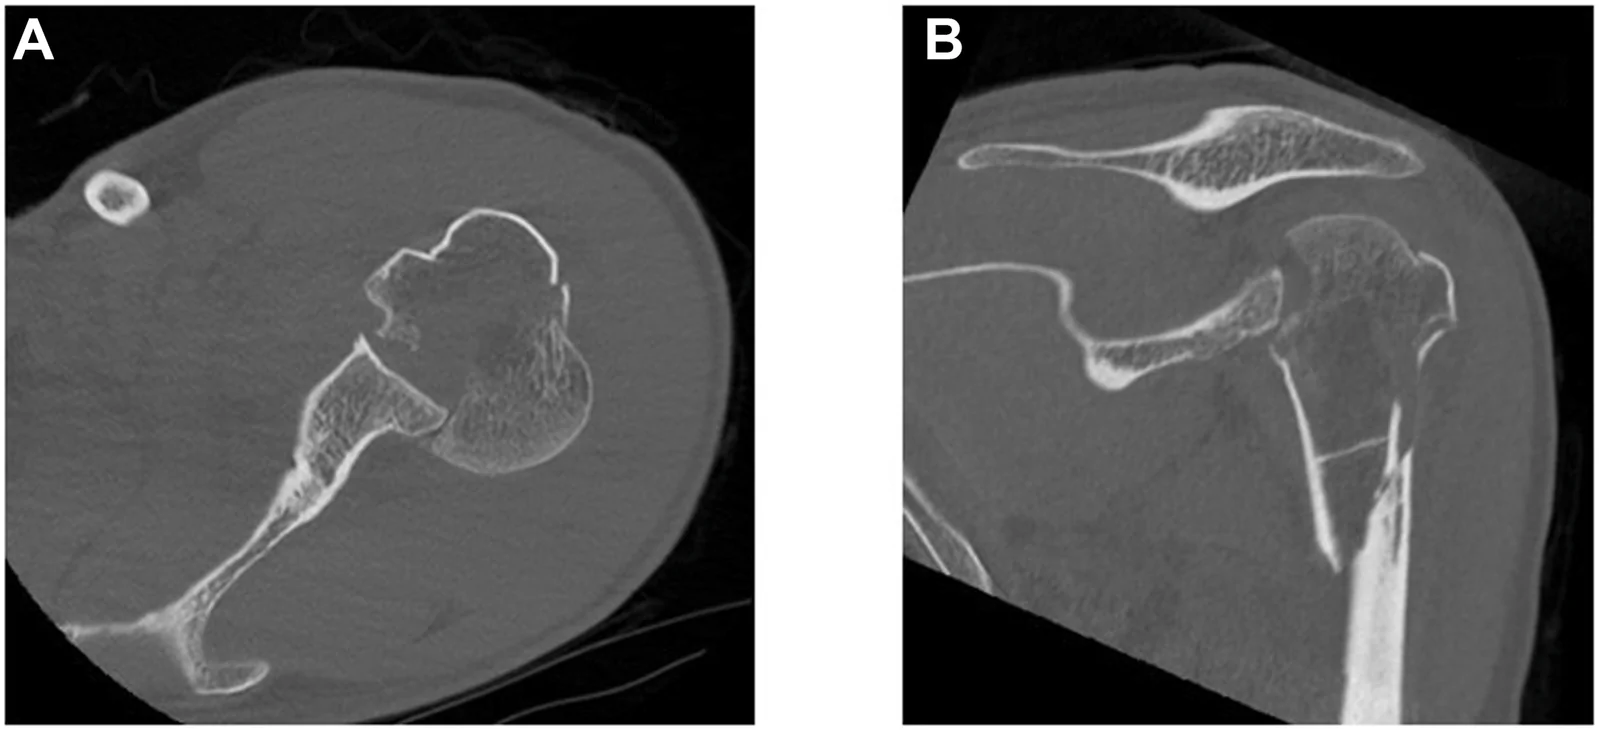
postoperative axial (A) and coronal (B) CT scan of the left shoulder showing a multifragmentary posterior fracture-dislocation of the proximal humerus. *CT*, computed tomography.
